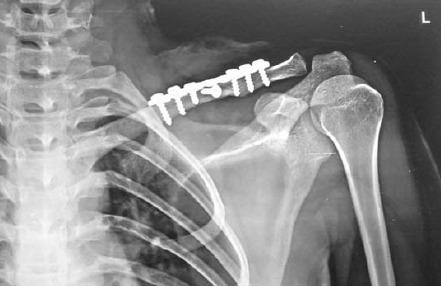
Fig. 3: Pre and Post op radiographs of left shoulder F3:
